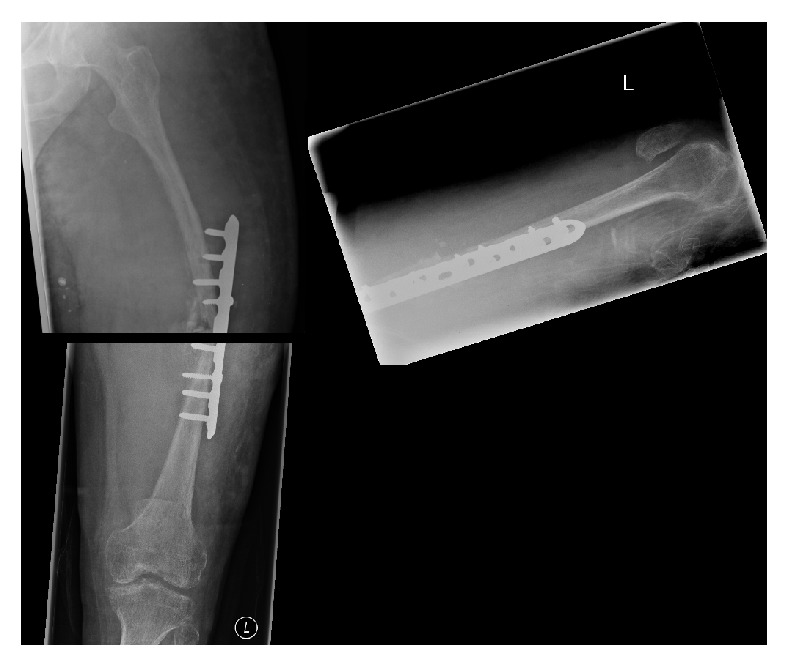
AP and lateral radiograph of the left femur immediately one month after open reduction, internal fixation.
